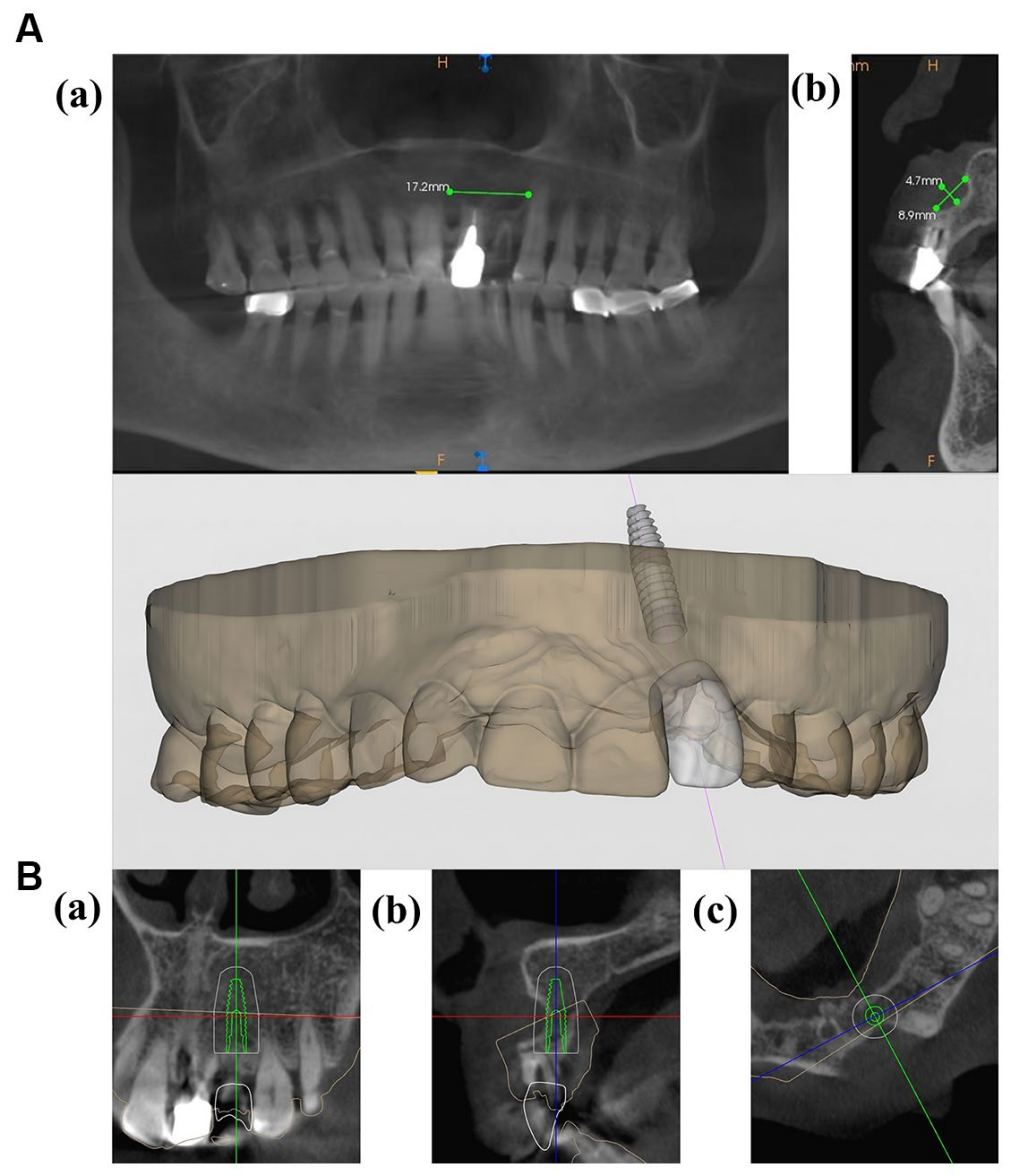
(A) (a,b) CBCT images of the patient before the operation. (B,C) Preoperative planning for implant placement.
